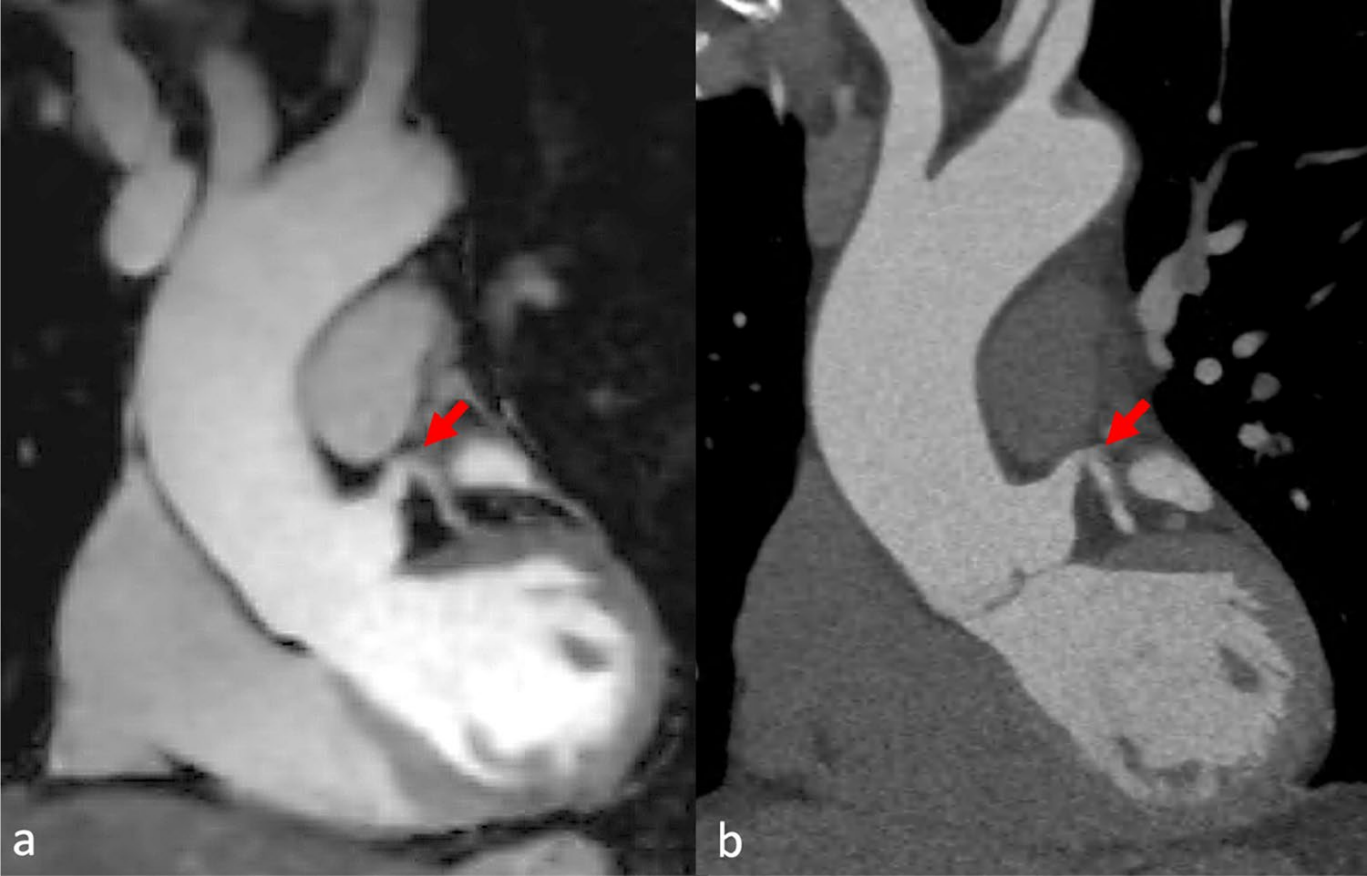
A patient had an unclear structure at the aortic root noted in the echocardiography. The initial CT scan was performed to confirm the findings, but it did not reveal any abnormalities. Coronal reformation of MRA (a) and CTA (b) of the aortic root and ascending aorta. Excellent depiction of the proximal left coronary artery (arrow)
